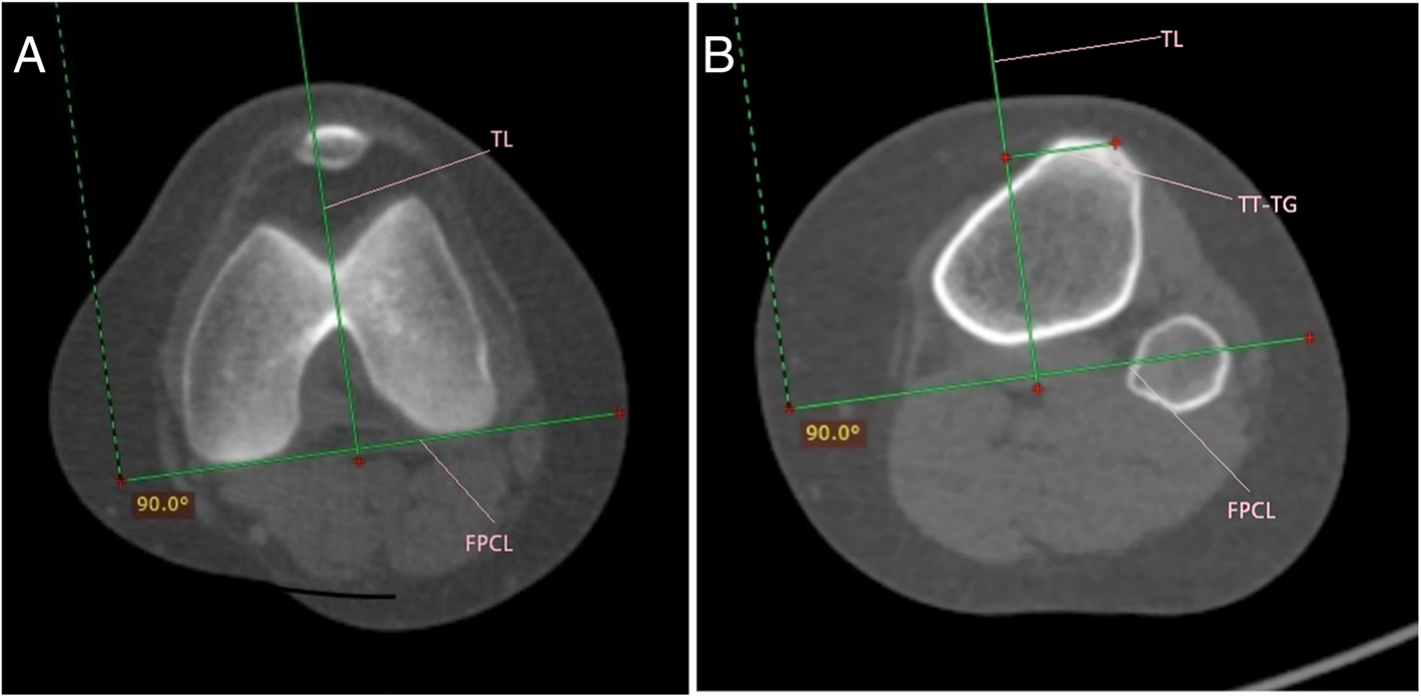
a, b: Technique for measuring TT-TG distance on Axial computed tomography. FPCL, femoral posterior condylar line; TL, trochlear line; TT-TG, tibial tubercle–trochlear groove distance
